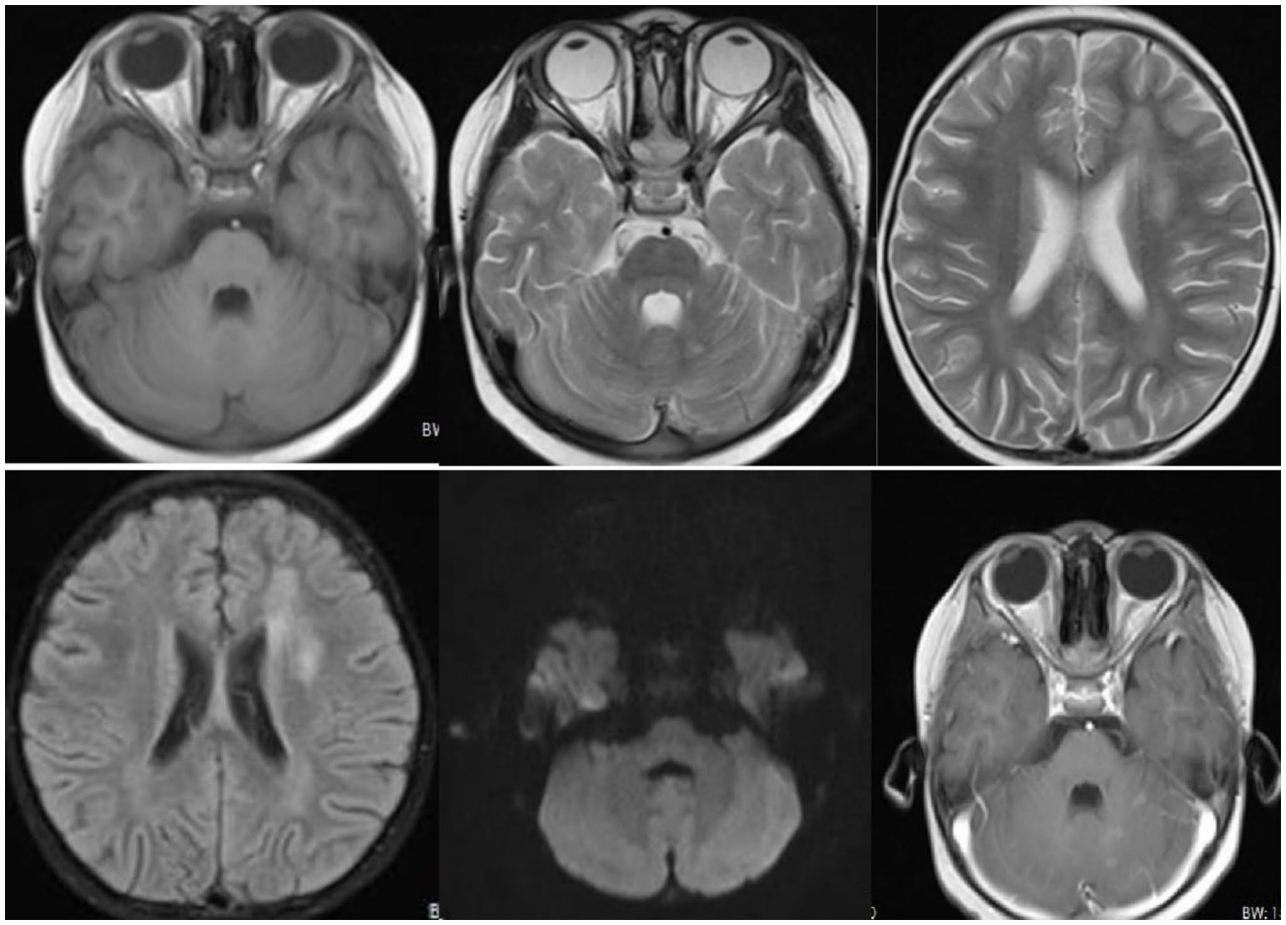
Radiological findings revealed patchy and slightly longer T1 and T2 signals with unclear boundaries and high signal intensity on T2-weighted fluid-attenuated inversion recovery (T2WI-FLAIR) in the right parietal lobe, left temporal lobe, and left lateral ventricular forefoot. The bilateral hippocampi demonstrated patchy, slightly longer T1 and T2 signals with unclear boundaries and high signal on T2WI-FLAIR. The left cerebellar hemisphere displayed multiple patchy and slightly longer T1 and T2 signals with clear boundaries and patchy enhancement on the contrast-enhanced scan. Long linear T1 and T2 signals were observed in the right parietal lobe, with linear enhancement seen on the contrast-enhanced scan.
